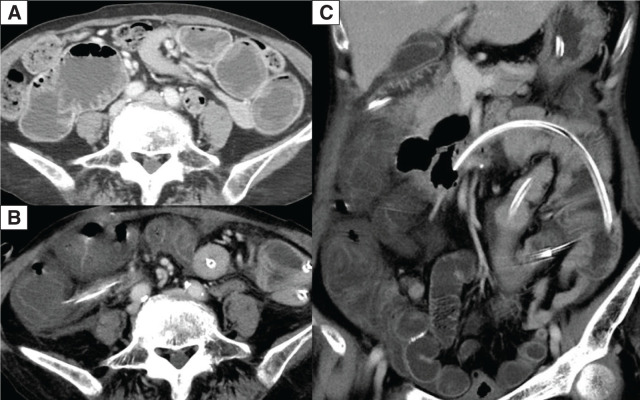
Contrast-enhanced CT images. (A) Axial image obtained at the disease onset, showing dilatation of the small intestine. (B, C) Preoperative images obtained during septic shock, showing that the colon is markedly edematous and slightly contrast-enhanced (B, axial view; C, coronal view).
